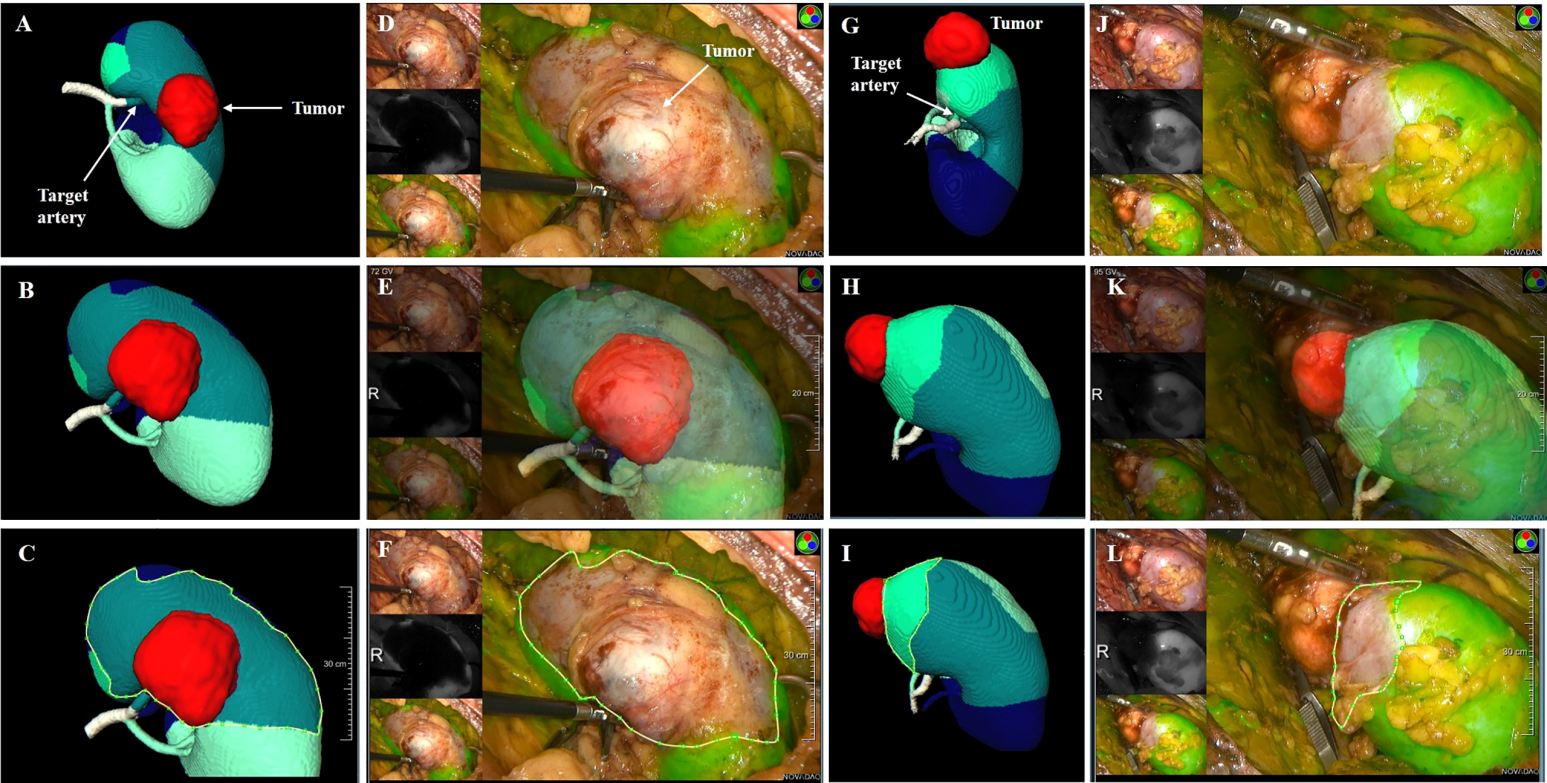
the clinical application of TKP model. (A–F) Case 1: a male patient with 3.8 cm tumor on the posterior part of the right kidney. The R.E.N.A.L score is 9. (G–L) Case 2: a female patient with 3.1 cm tumor on the upper polar of the right kidney. The R.E.N.A.L score is 5. (A, G) The TKP model is automatically established based on contrast CT scan and the target segmental artery is determined. (D–F, J–L) ICG is injected immediately after clamping, and the absence of perfusion on the renal parenchyma was confirmed with NIRF imaging. (C vs. F, I vs. L) The algorithm of dice coefficient is applied in assessing the similarity, and the dice coefficient is 0.92 and 0.81, which indicates that the ischemic region recorded in NIRF imaging is highly consistent with the perfusion region predicted in the TKP model.
